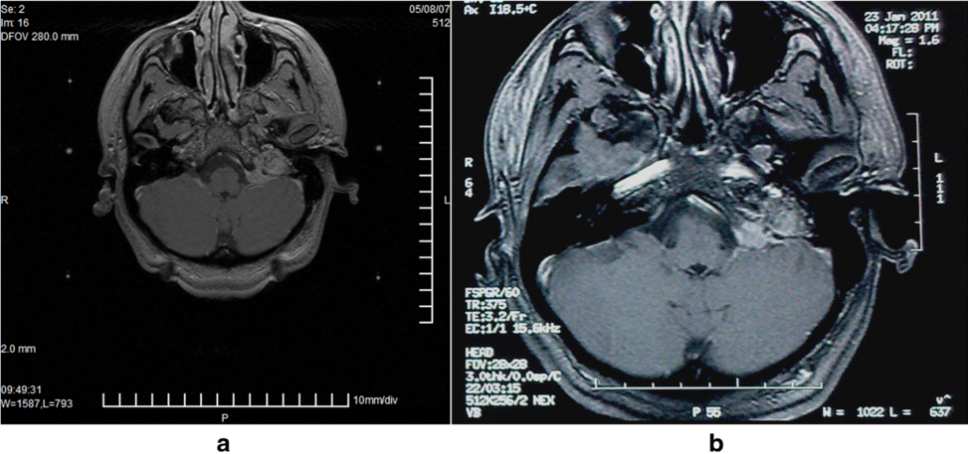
a Pre-gamma knife stereotactic contrast-enhanced MR image of a patient with a large 6 cm^3^ left glomus jugulare treated with 15 Gy marginal dose to 35 % isodose line with 97 % coverage with the prescribed dose. b Contrast-enhanced MR image of the same patient 72 months after GKS showing some tumor regression
